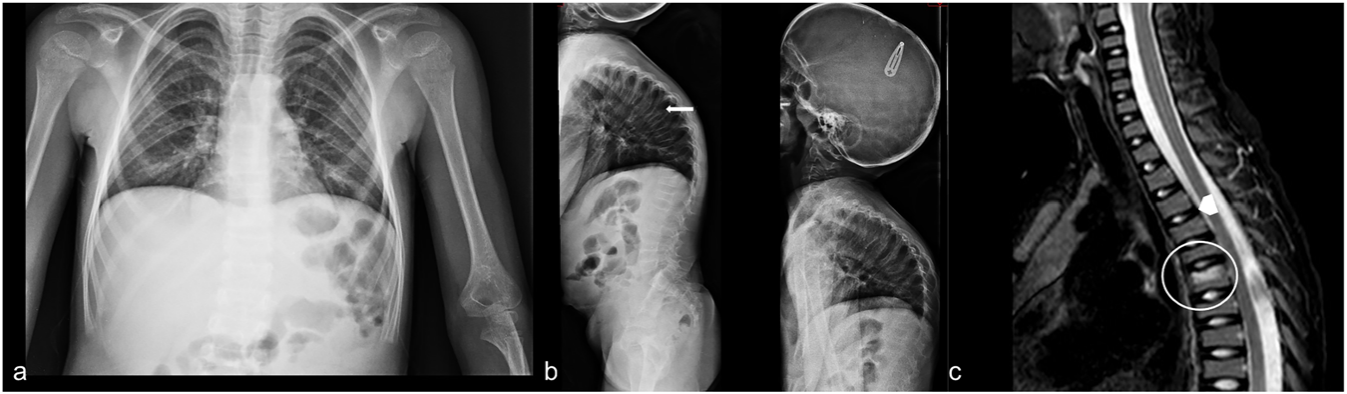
Patient affected by osteogenesis imperfecta. X-ray pictures show diffuse alteration of the vertebral bodies with a reduction in height (platyspondyly) and depression of the upper side of vertebral bodies—namely at the thoracic level (white arrow, b); marked gibbous deformation of the dorsal spine (b). Mildly dysmorphic appearance of the rib cage (a). MRI shows an area of signal hyperintensity in T2/STIR of the soma of Th6 due to edema (possible hyperemia or microfracture) (c, white circle). The intervertebral discs take an oval appearance in the absence of significant protrusions (c, white arrowhead)
